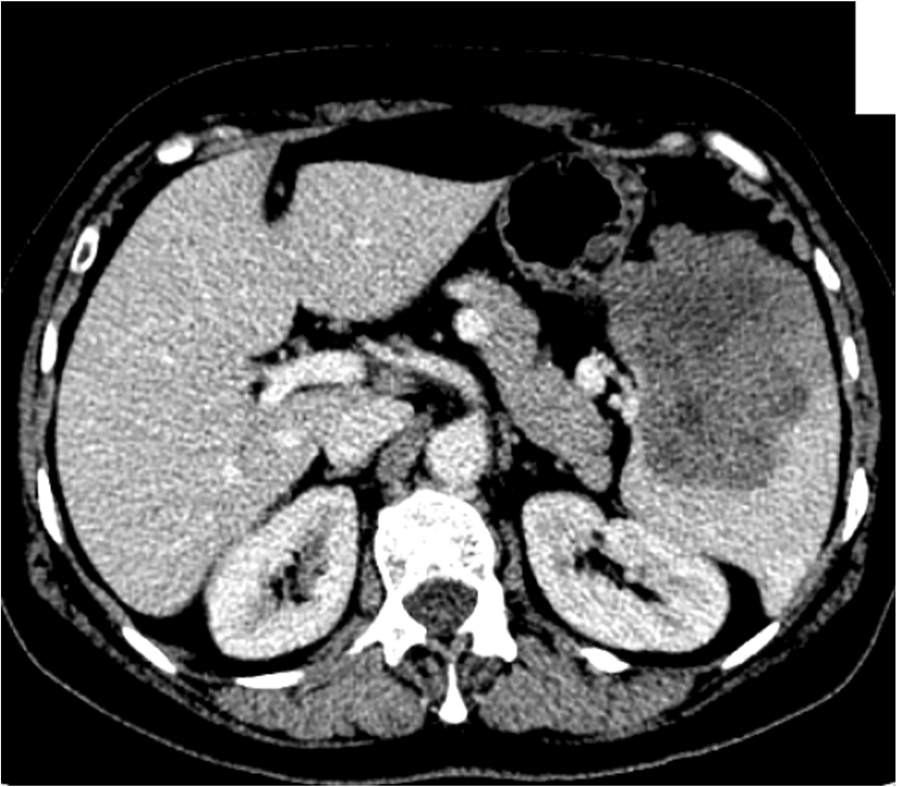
Abdominal computed tomography. Abdominal computed tomography revealed a heterogeneous hypodense mass of the spleen with suspected necrotic changes.
